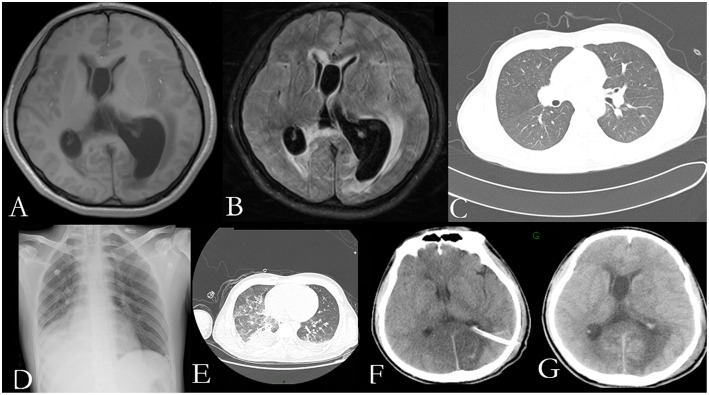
Radiological examination of the patient: T1 weighted axial and T2-flair brain MR imaging showing pachymeningitis and hydrocephalus (A,B); The lung CT on the first day of his second admission shows no obvious signs of inflection (C); The chest radiograph (D); and lung CT (E) show pulmonary infection, pleural effusion, and partial atelectasis; Head CT on the first day after surgery (F) and a week after surgery (G) show rapid accumulation of cerebrospinal fluid (CSF).
